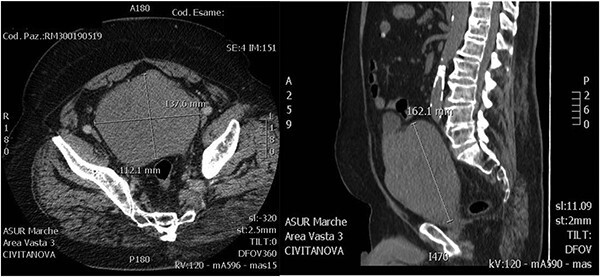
Axial and sagittal CT images.
